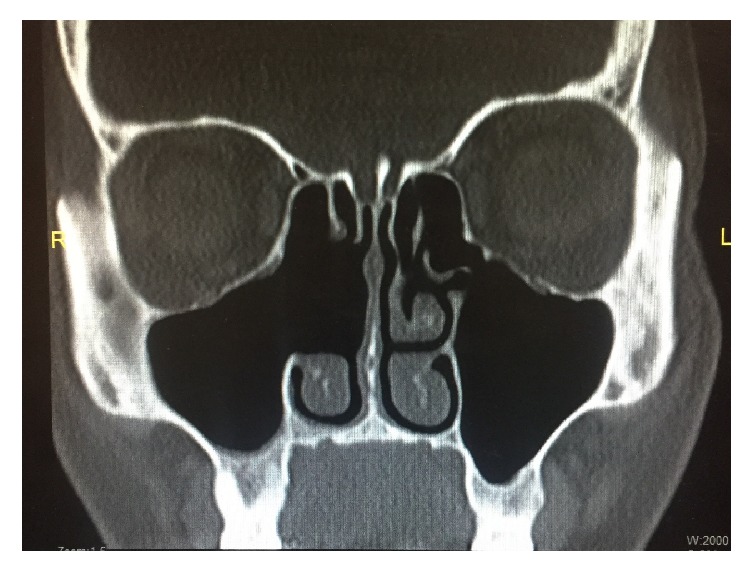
A postoperative coronal CT image demonstrating well-aerated right maxillary and ethmoid sinuses without mucosal thickening with a patent ostiomeatal complex after right maxillary antrostomy, middle turbinectomy, and anterior and posterior ethmoidectomy.
